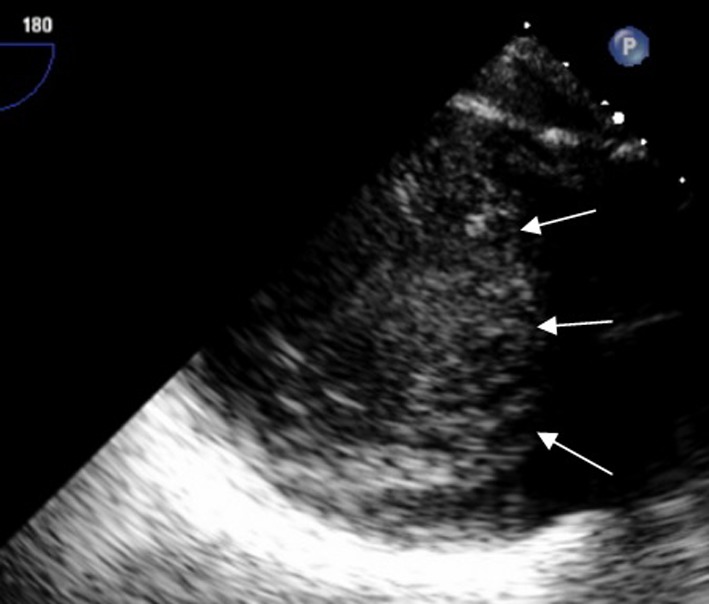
Transesophageal echocardiogram with trans gastric view with contrast demonstrates the contrast filling in the inter-trabecular recesses within the ventricular cavity.
